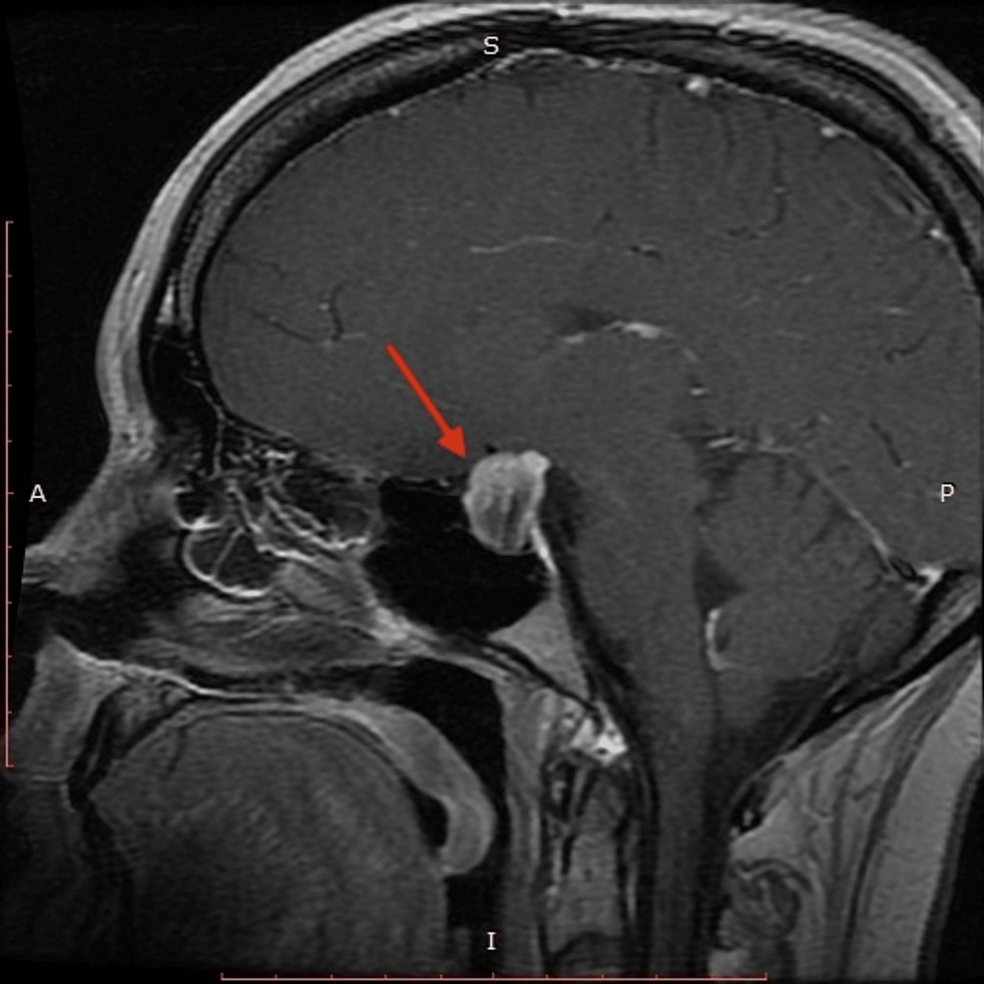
MRI of pituitary fossa compressing the optic chiasm
